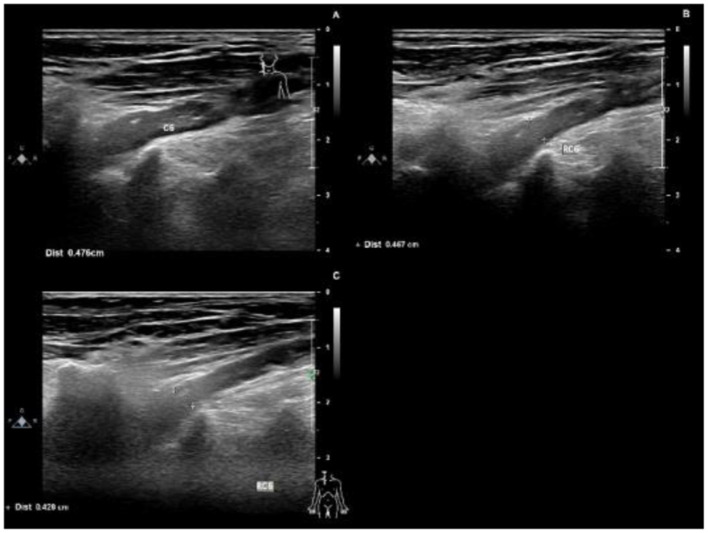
Ultrasounds 4 months after the onset of the disease, the right C6 nerve root was thickened, with a thickness of 0.476 cm (A). 6 months after the onset of the disease, the right C6 nerve root was thickened, with a thickness of 0.467 cm (B). 11 months after the onset of the disease, the right C6 nerve root was thickened, with a thickness of 0.426 cm (C).
